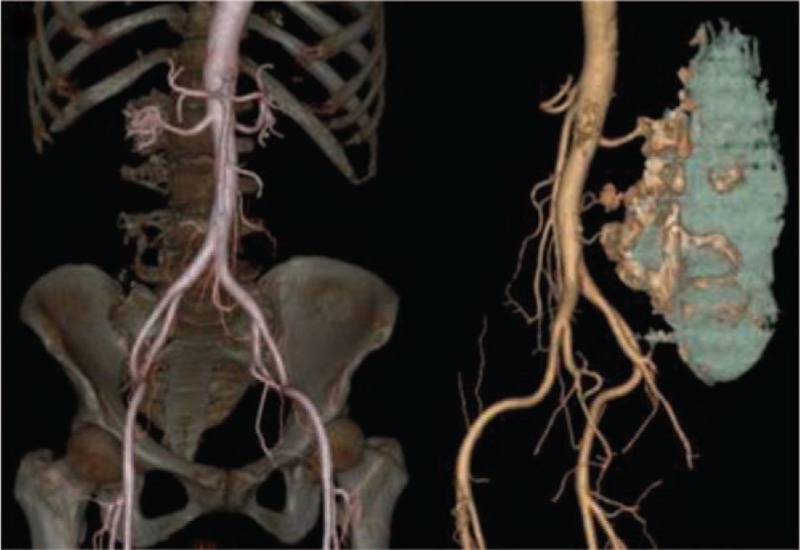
Abdominal aorta computed tomography angiography examination showed that 2 and 3 right lumbar arteries were on the lesion surface, with some small branches entering the lesion.
